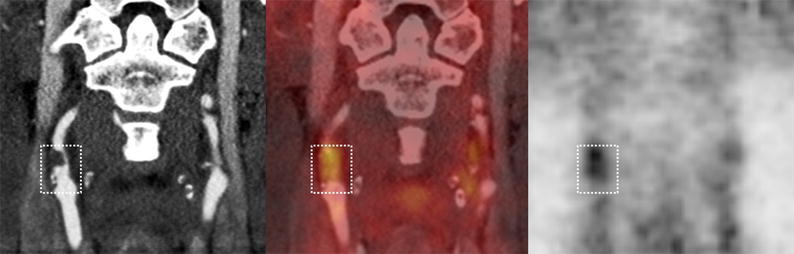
^18^F-FDG PET/CT of stenotic plaque in the right internal carotid artery. From left to right: CT angiography, co-registered PET/CT, and ^18^F-FDG PET. The white box shows the plaque extension craniocaudally with lumen contrast-filling defect and vessel wall thickening on CT angiography (left image)
